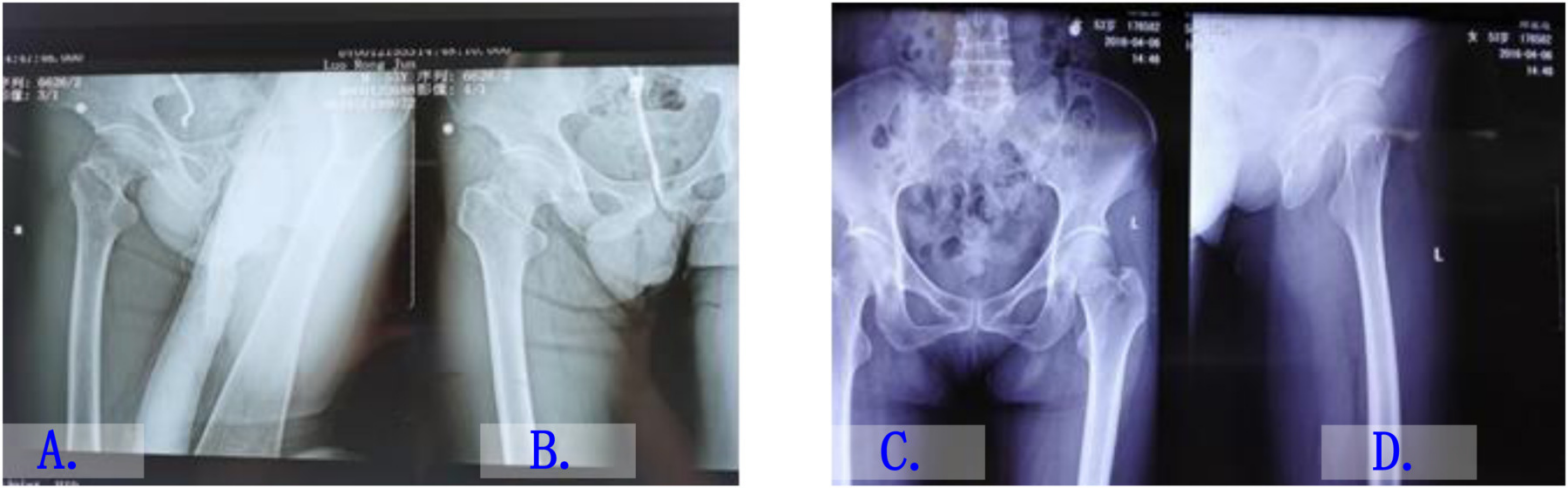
Imaging examination images of typical cases. (A) Preoperative x-ray of a patient with a right femoral neck fracture. (B) Postoperative x-ray after fixation with a locking compression plate. (C) Preoperative x-ray of a patient with a left femoral neck fracture. (D) Postoperative x-ray after fixation with cannulated screws.
